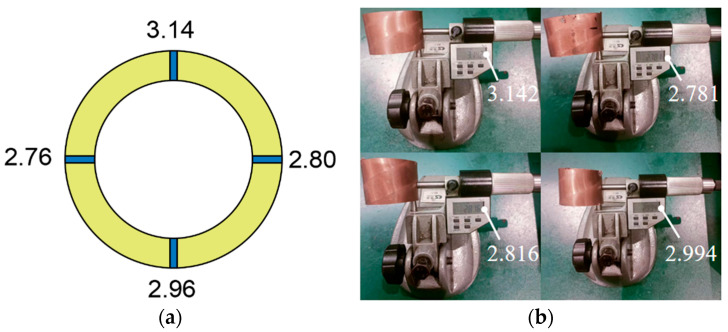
Comparison of wall thickness data: (a) Ultrasonic testing data; (b) Hand measurement data.
